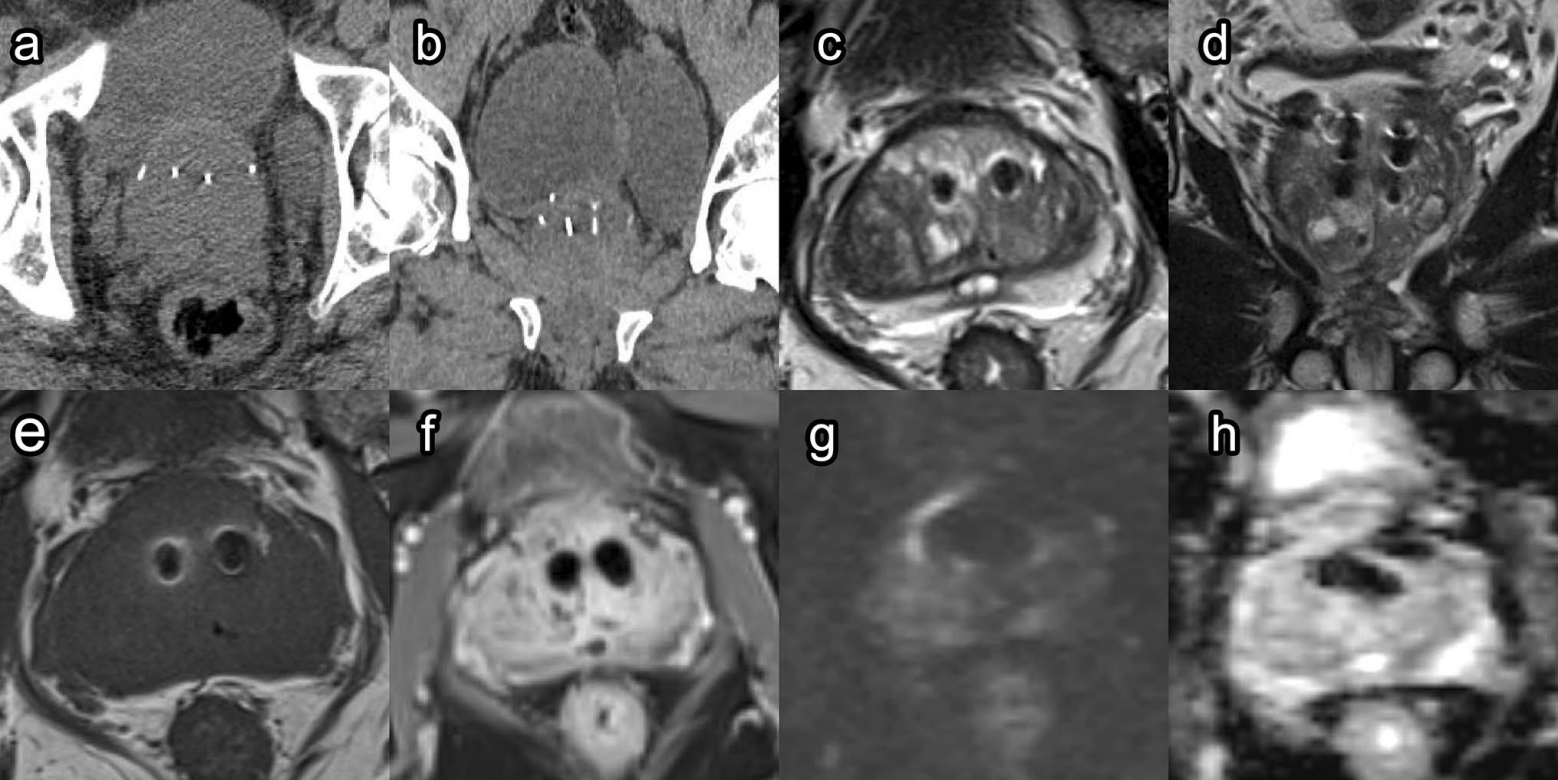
Prostatic urethral lift. Post-prostatic urethral lift prostate CT: Axial (a) and coronal (b) images of a patient with Urolift® implant. Metallic attenuation objects are noted in appropriate position along the bilateral median lobes. Post-prostatic urethral lift prostate MRI: Axial T2 (c), coronal T2 (d), pre-contrast T1 (e), and post-contrast T1 (f) images demonstrate a metal susceptibility artifact causing a signal void with peripheral hyperintensity in the region of the implant. DWI at B1400 (g) and ADC (h) images demonstrate exaggerated signal void in the region of the implant
